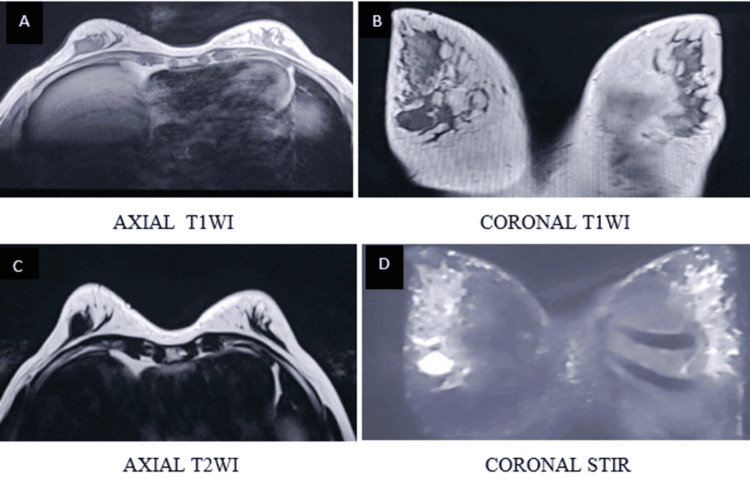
A well-defined oval-shaped mass lesion in the lower outer quadrant of the right breast appears isointense on axial T1WI (A, B), isointense on axial T2WI (C), and hyperintense on coronal STIR images (D) T1WI: T1-weighted image; T2WI: T2-weighted image; STIR: short TI inversion recovery
